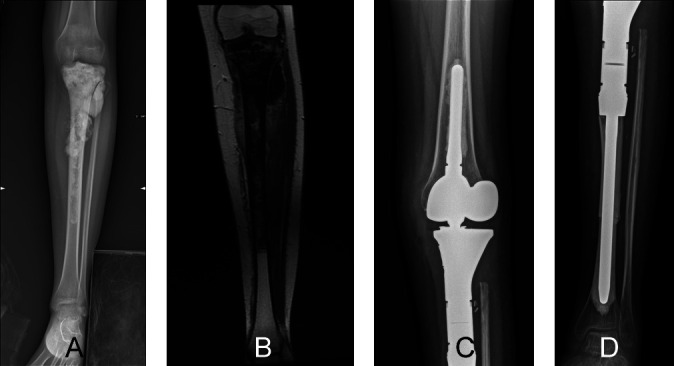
A 17-year-old girl diagnosed with osteosarcoma of the left proximal tibia who was treated by osteoarticular resection and modular prosthesis reconstruction. (A) Preoperative anteroposterior radiographic view showing a permeating sclerotic lesion of the proximal tibia. (B) A coronal-T1 magnetic resonance image showing the tumor located at the proximal tibia that extends to the diaphysis. Postoperative radiographs (C and D) show modular prosthesis reconstruction and intercalary massive bone allograft augmentation of the proximal tibia at the 12-month follow-up. The modular prosthesis was one of the treatments of choice in children at the end of their skeletal growth undergoing joint resection for bone sarcoma.
